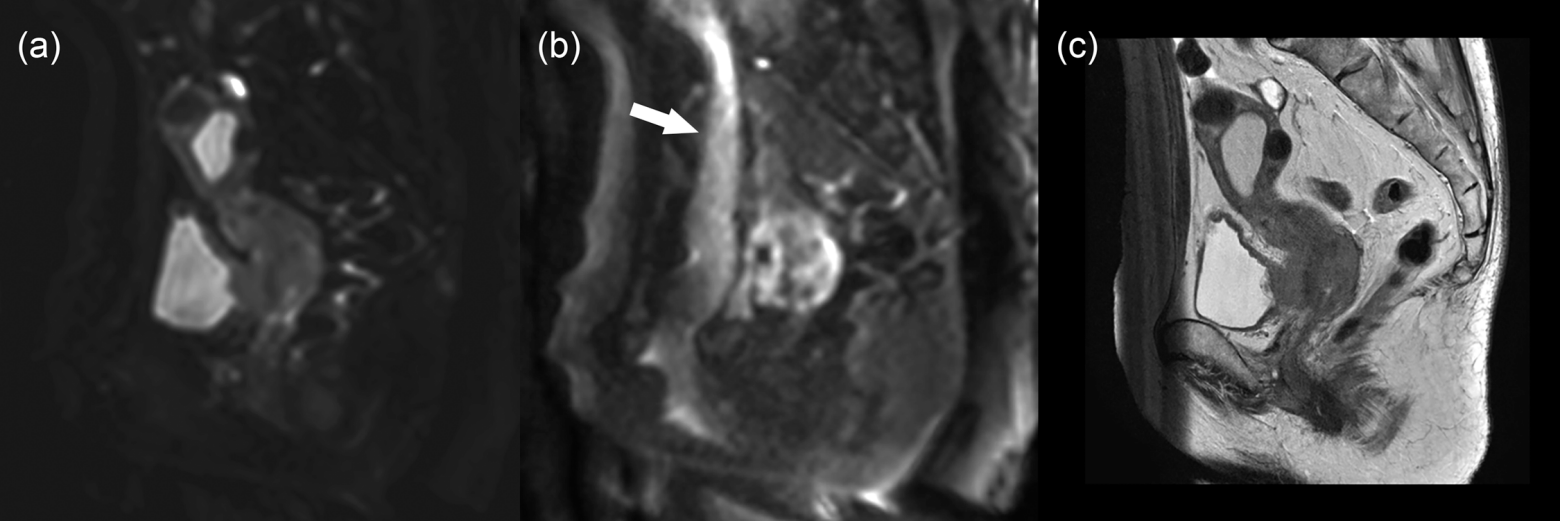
A 67-year-old woman with squamous cell carcinoma (keratinizing type) of the uterine cervix. (a) Sagittal diffusion-weighted image at *b* = 0 s/mm^2^, (b) sagittal diffusion-weighted image at *b* = 2500 s/mm^2^, and (c) sagittal T2-weighted image show the tumor in the uterine cervix. Because of a severe ghost artifact from the abdominal wall (arrow), it was hard to calculate diffusivity and kurtosis values for the myometrium. However, those values could be calculated for the cervical tumor because of the lesser degree of artifacts of the uterine cervix.
